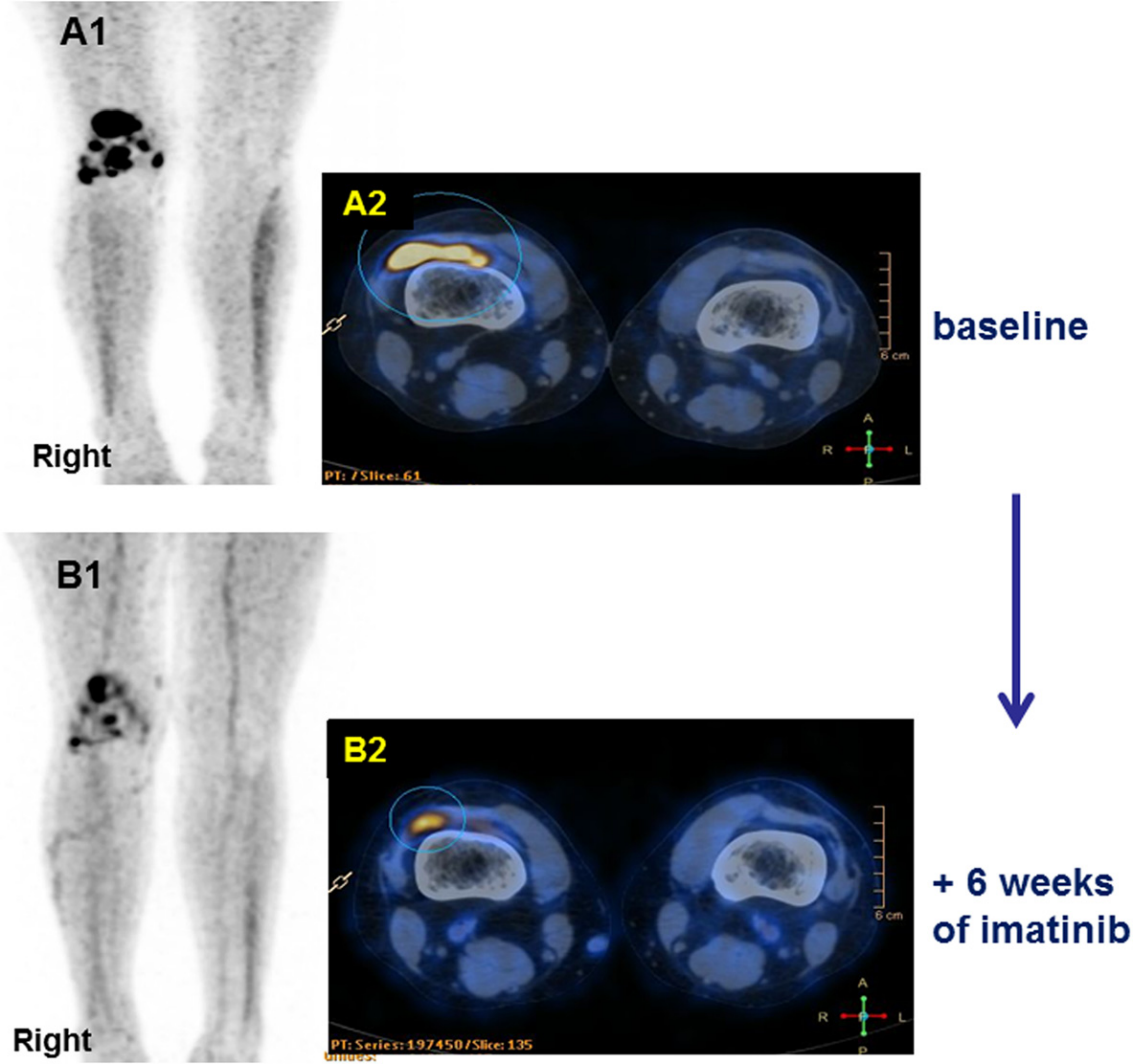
[18F]fluorodeoxyglucose (FDG) PET/CT tumor assessment of Patient 2. Baseline PET/CT maximum projection image (MIP) (panel A1) showed abnormal FDG focal uptakes in the right knee PVN with a SUVmax of 14.7 g/ml, as detailed by fused PET/CT transaxial slice (panel A2). After 6 weeks of treatment, PET/CT MIP (panel B1) and fused PET/CT transaxial slice (panel B2) showed a marked decrease of tumor FDG uptake (SUVmax 4.1 g/ml, i.e. 72% reduction).
